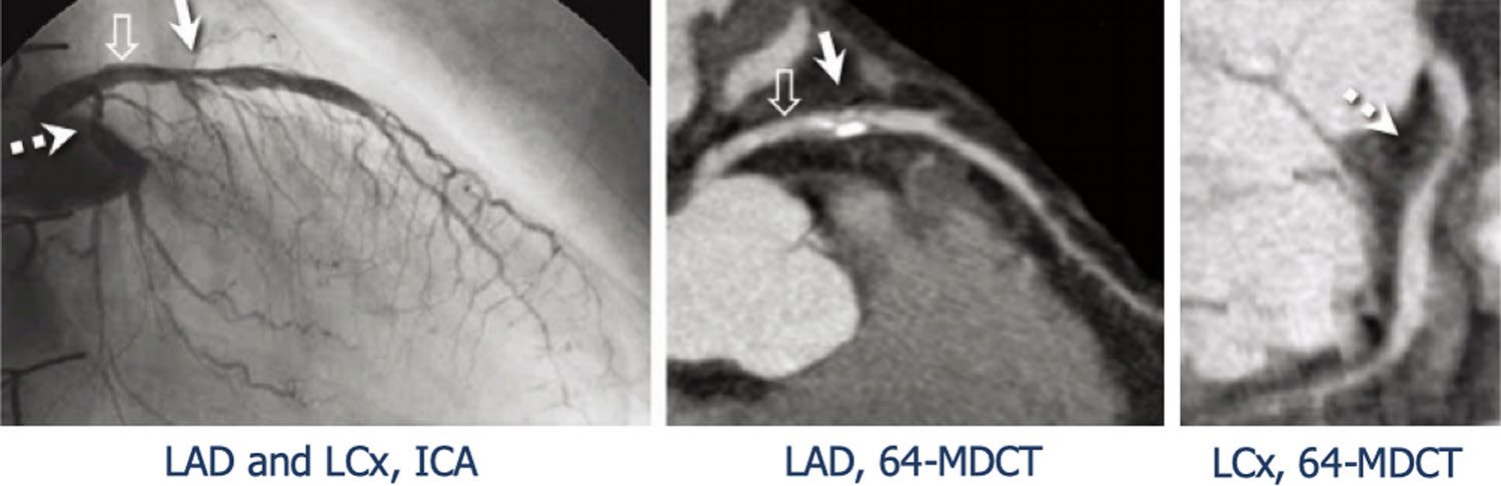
Invasive coronary angiography vs coronary computed tomography angiography. Presence of coronary allograft stenosis in proximal-middle left anterior descending artery (LAD, continuous arrows) and proximal circumflex artery (LCx, dashed arrow), as detected by either invasive coronary angiography (ICA) and 64-slice multidetector computed tomography (MDCT). ICA, invasive coronary angiography; LAD, left anterior descending artery; LCx, left circumflex artery; MDCT, multidetector computed tomography. Adapted from Nunoda S et al., 2010 (10.1253/circj.cj-09–0800) [84]
